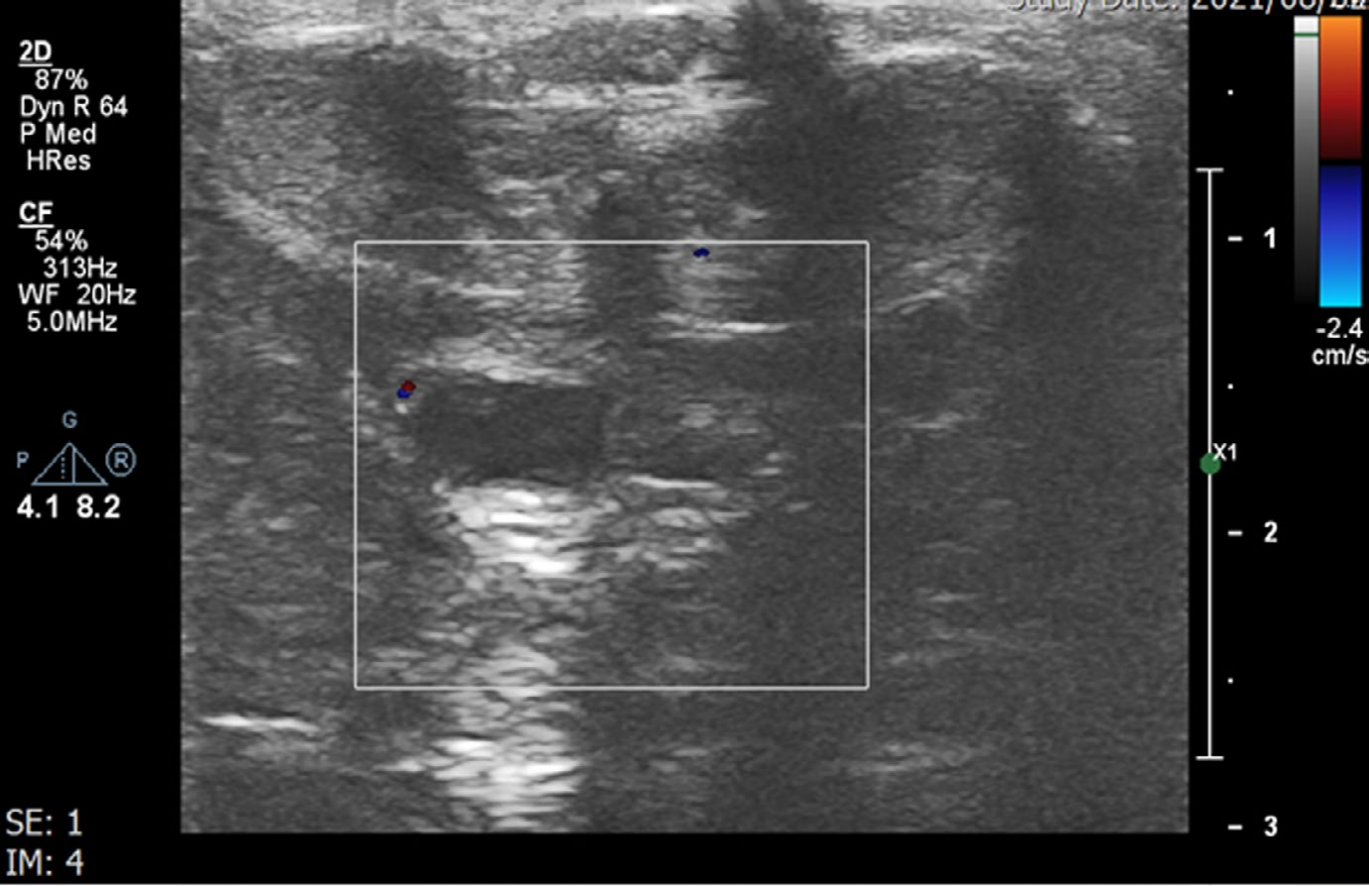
Ultrasound evaluation of deep dorsal penile vein (Arrow) shows no color in the vein. The vein contains echogenic thrombosis. White Stars: Normal right and left corpus cavernosum. Black star:Normal corpus spongiosum
